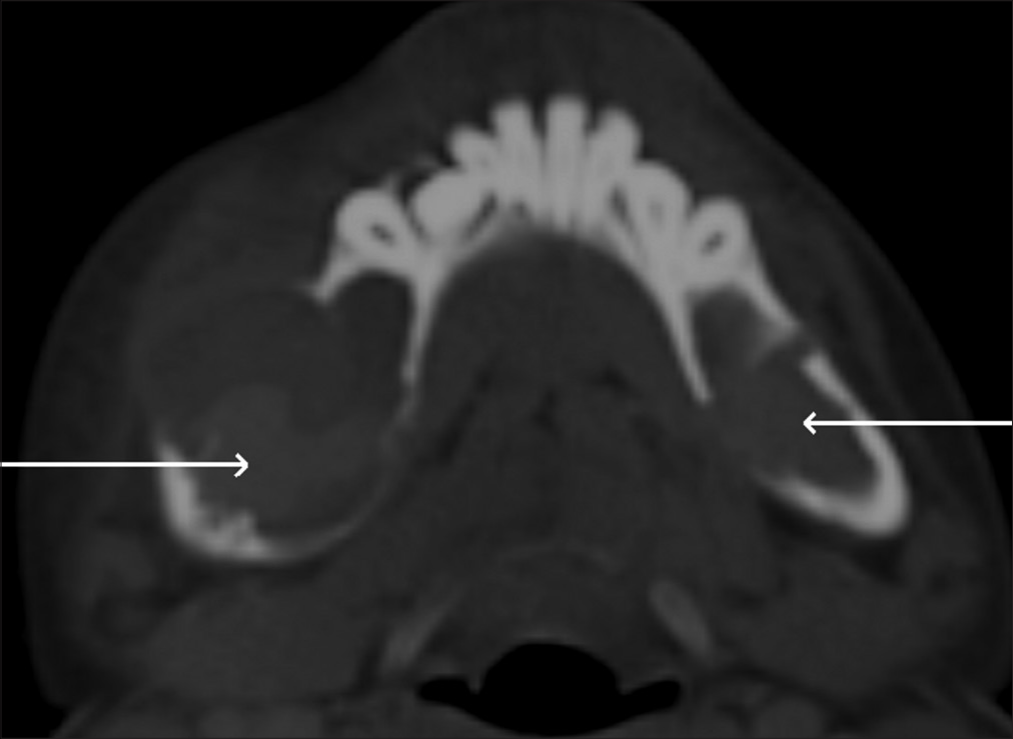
Axial, non-enhanced CT scan shows the expansile and osteolytic nature of the lesion, with hyperdense areas within (arrows) signifying partial thrombosis
